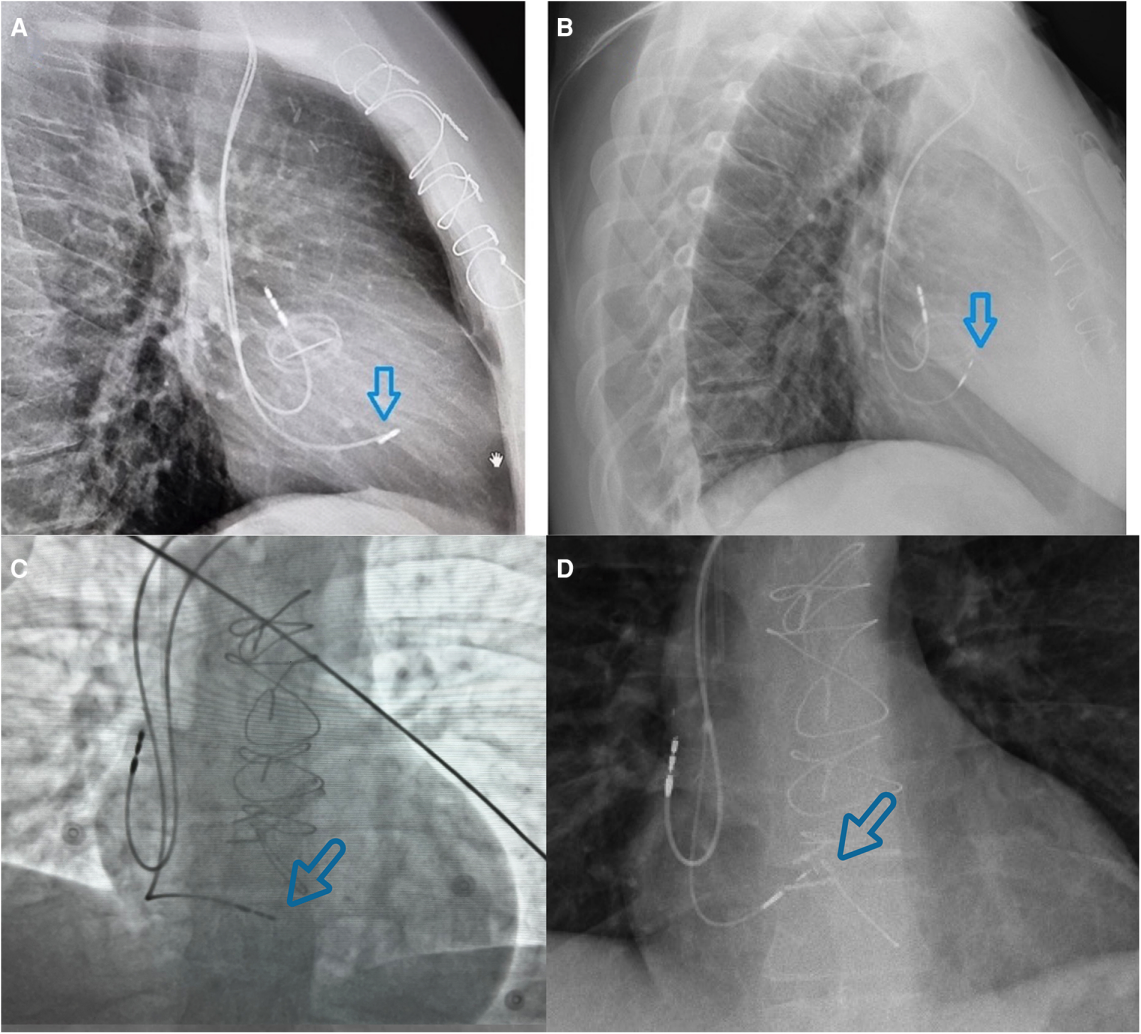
Different views to compare lead position in left bundle branch area pacing (left images) and at the area of the His bundle (right images). (A) Lateral view chest x-ray image of the ventricular lead positioned in the LBBAP area. (B) Lateral view chest x-ray image of the ventricular lead in the area of the His bundle (thinner lumenless lead; approximately 2 cm higher than the LBBAP lead). (C) Fluoroscopic antero-posterior view of the ventricular lead positioned in the LBBAP area. (D) Antero-posterior x-ray view of the ventricular lead in the area of the His bundle.
